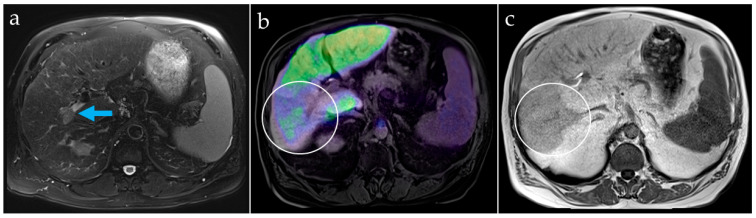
Patient B. Contrast-enhanced MRI of the liver in patient B shows tumor involvement and thrombus in the right portal vein branch ((a), arrow). The PET/MR fusion image (b) reveals liver areas with reduced [^68^Ga]Ga-BP-IDA uptake, corresponding to areas with liver texture disturbance on MRI (c), attributable to liver function impairment larger than the HCC lesions (white circles).
